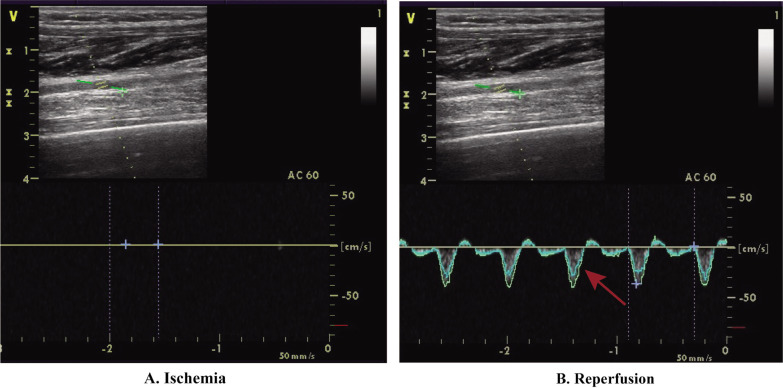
Doppler ultrasonography.
